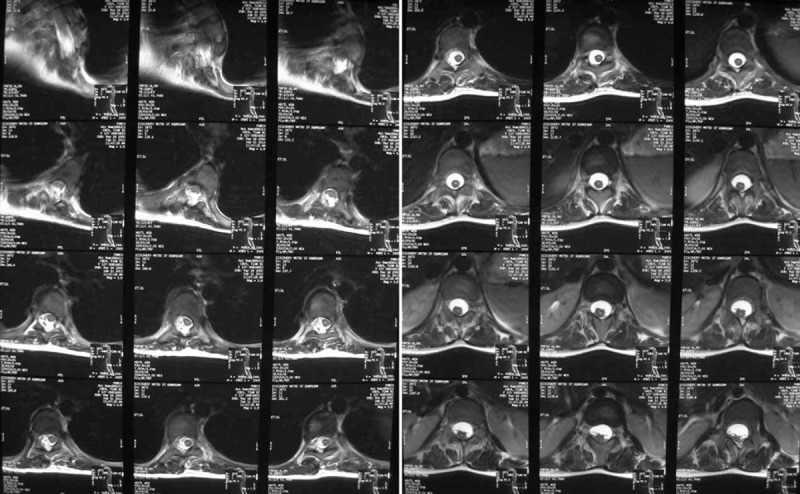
Magnetic resonance imaging (MRI) revealed syringomyelia.
